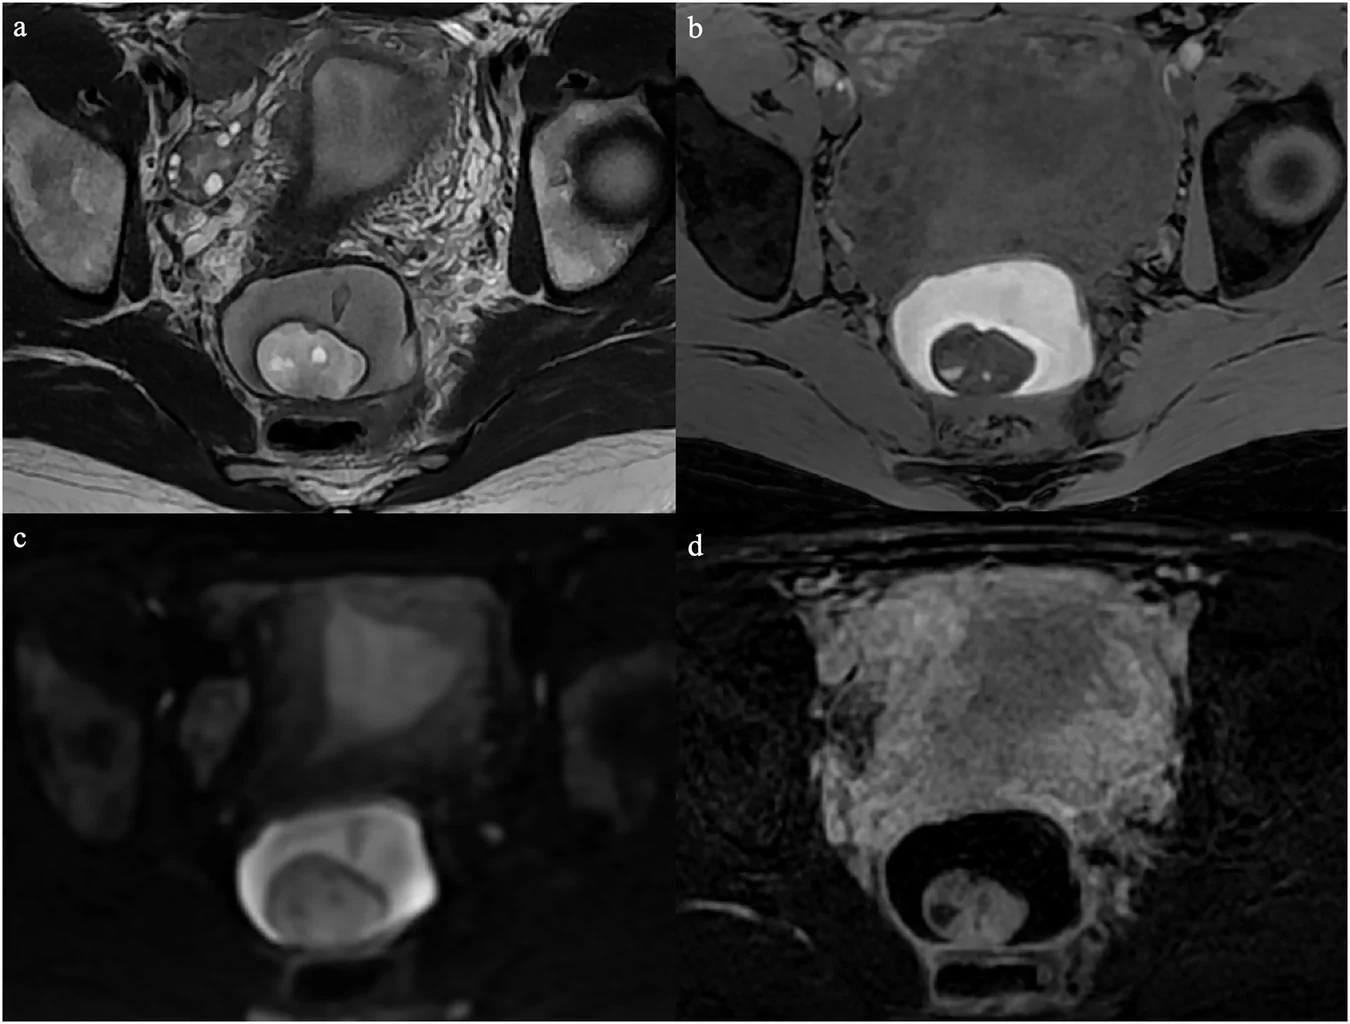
Endometriotic polyp. a Axial T2-weighted sequence; b axial T1 water-only image from a T1-weighted Dixon sequence; c DWI sequence; d postcontrast fat-saturated T1-weighted sequence. The images show a posterior pelvic cyst with endometriotic liquid that contains a solid component with high T2W signal and low DWI signal. Injection shows enhancement of the solid part. TIC from DCE MR sequence (not presented here) shows an intermediate-risk time-intensity curve. Thus, the lesion was classified as O-RADS MR score 4. This case highlights the usefulness of subtracted images
